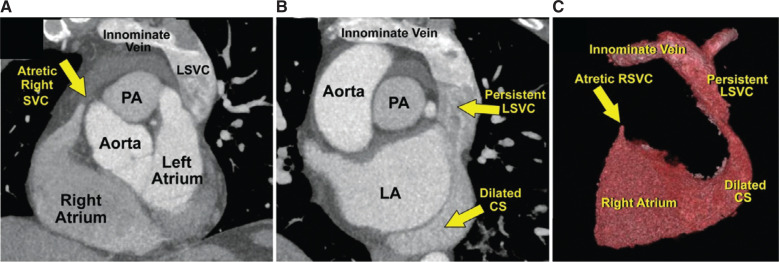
A: Computed tomography shows an atretic right superior vena cava (SVC) and left SVC connected to the innominate vein. B: Persistent left SVC draining into a dilated coronary sinus is seen. C: Three-dimensional reconstruction of a persistent left SVC and dilated coronary sinus is shown. *Abbreviations:* CS, coronary sinus; LA, left atrium; LSVC, left superior vena cava; PA, pulmonary artery; RSVC, right superior vena cava; SVC, superior vena cava.
